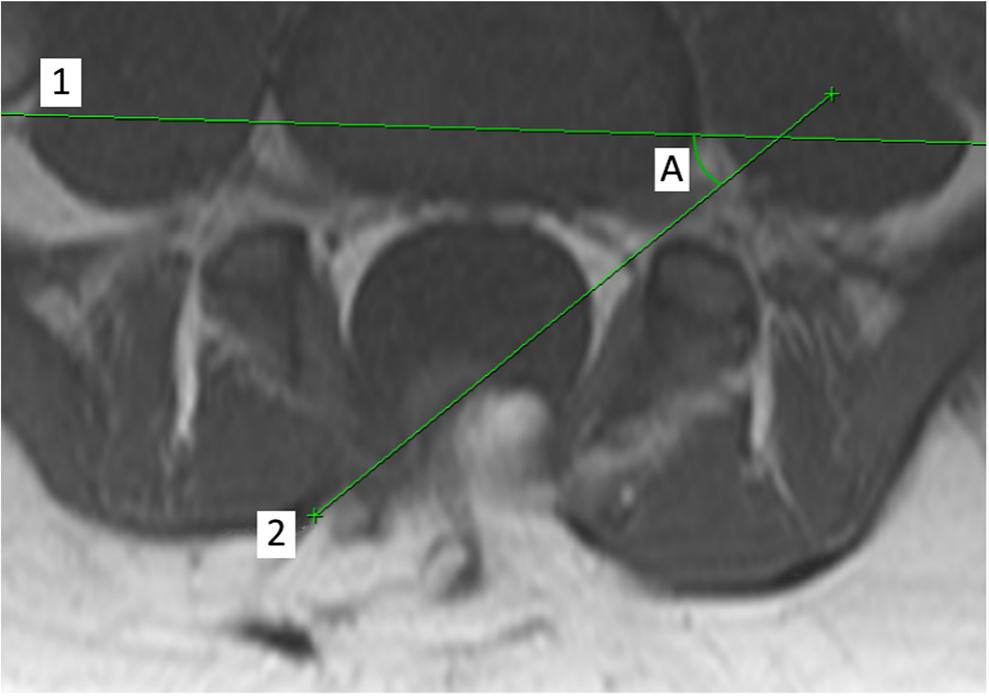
Measurement of rotation on T1 axial MR imaging with lipoma-neural placode rotated to the left. Lines that are drawn through coronal plane of vertebral body (1) and coronal plane of lipoma-neural placode at maximal rotation (2). Degree of rotation (A) measured as angle subtended by the plane of the placode (2) and horizontal plane (1)
